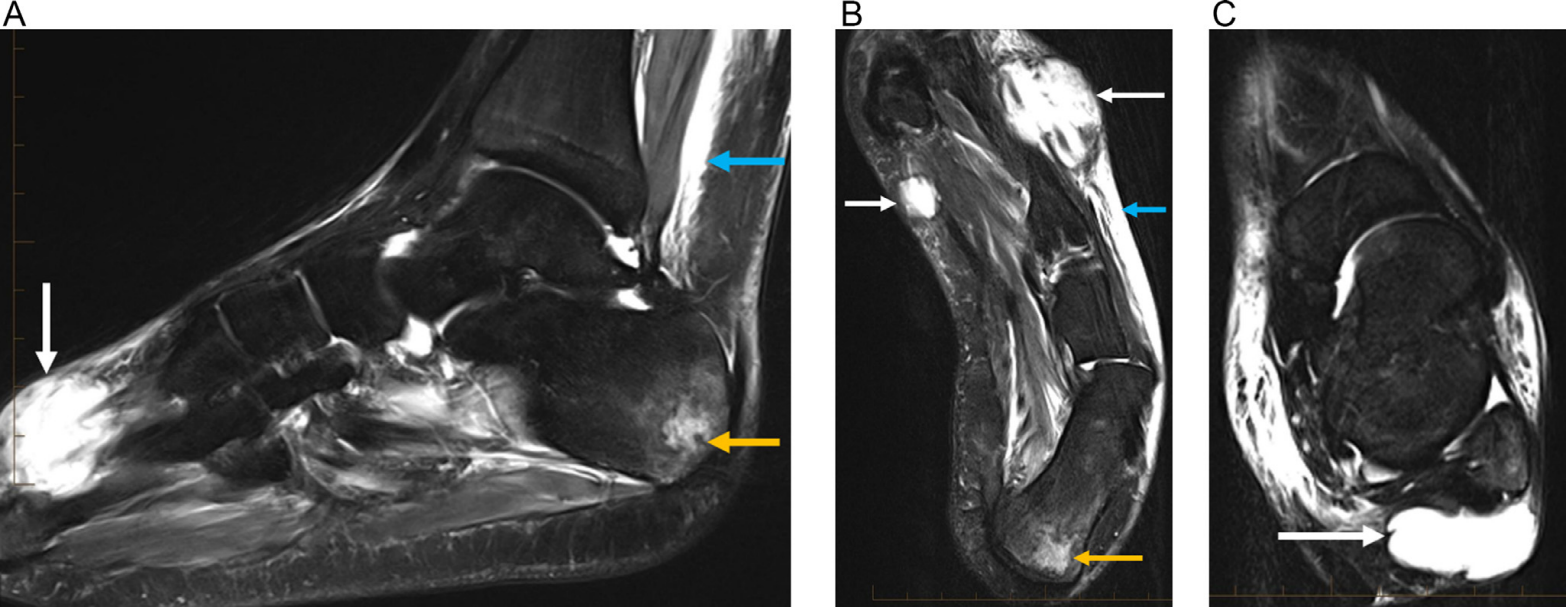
(A) Sagittal T2 fat sat image from baseline MRI of the left foot shows focal hyperintensity within the posterior calcaneus with surrounding ill-defined marrow edema pattern (orange arrow). This is not associated with the Achilles tendon or plantar fascia attachments. A focal fluid collection is seen in the dorsal soft tissues of the forefoot with traversing extensor tendons (white arrow). Edema is noted along Kager's fat pad (blue arrow). (B) Axial T2 fat sat image from baseline MRI of the left foot confirms focal marrow signal abnormality in the posterior calcaneus (orange arrow). Fluid collections are seen in the forefoot medially and laterally (white arrows). Subcutaneous edema is present laterally (blue arrow). (C) Axial T2 fat sat image from baseline MRI of the left foot demonstrates a focal fluid collection posterior to the lateral malleolus (arrow). Diffuse subcutaneous edema is noted (Color version of the figure is available online.)
